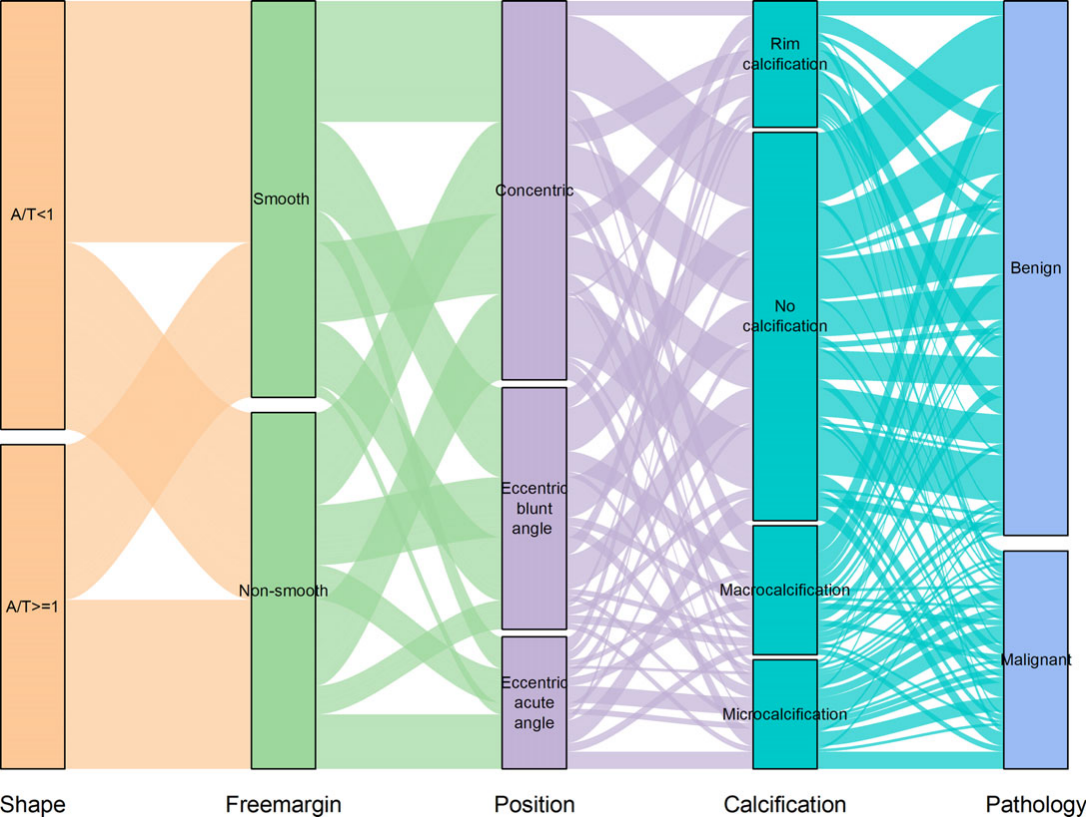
The distributions of all PCTNs in the primary cohort in the ultrasound features shows as Sankey plot.
